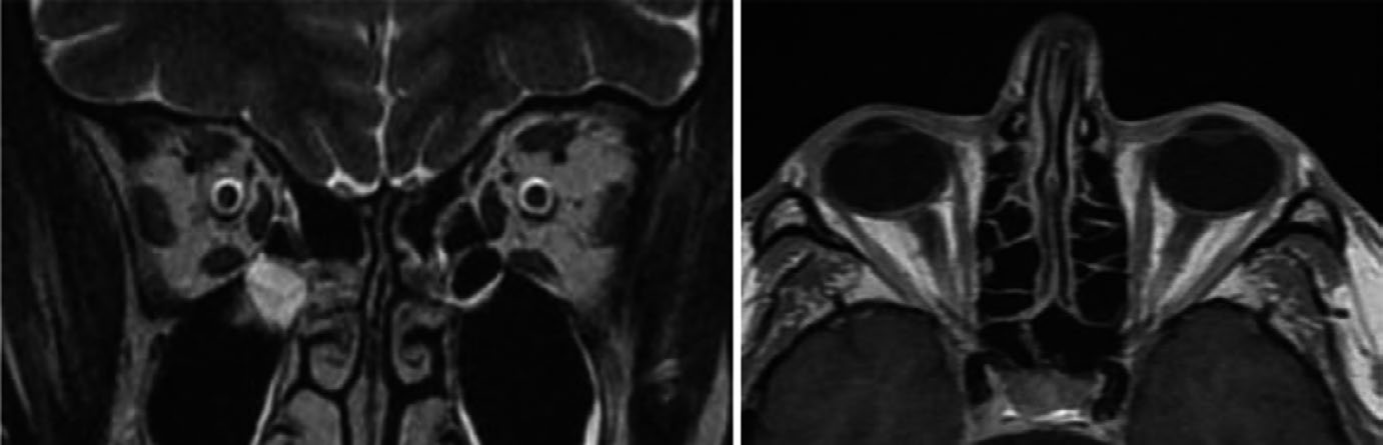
Coronal (A) and axial (B) T2 MRI images of the optic nerves showing no evidence of brain infiltration
